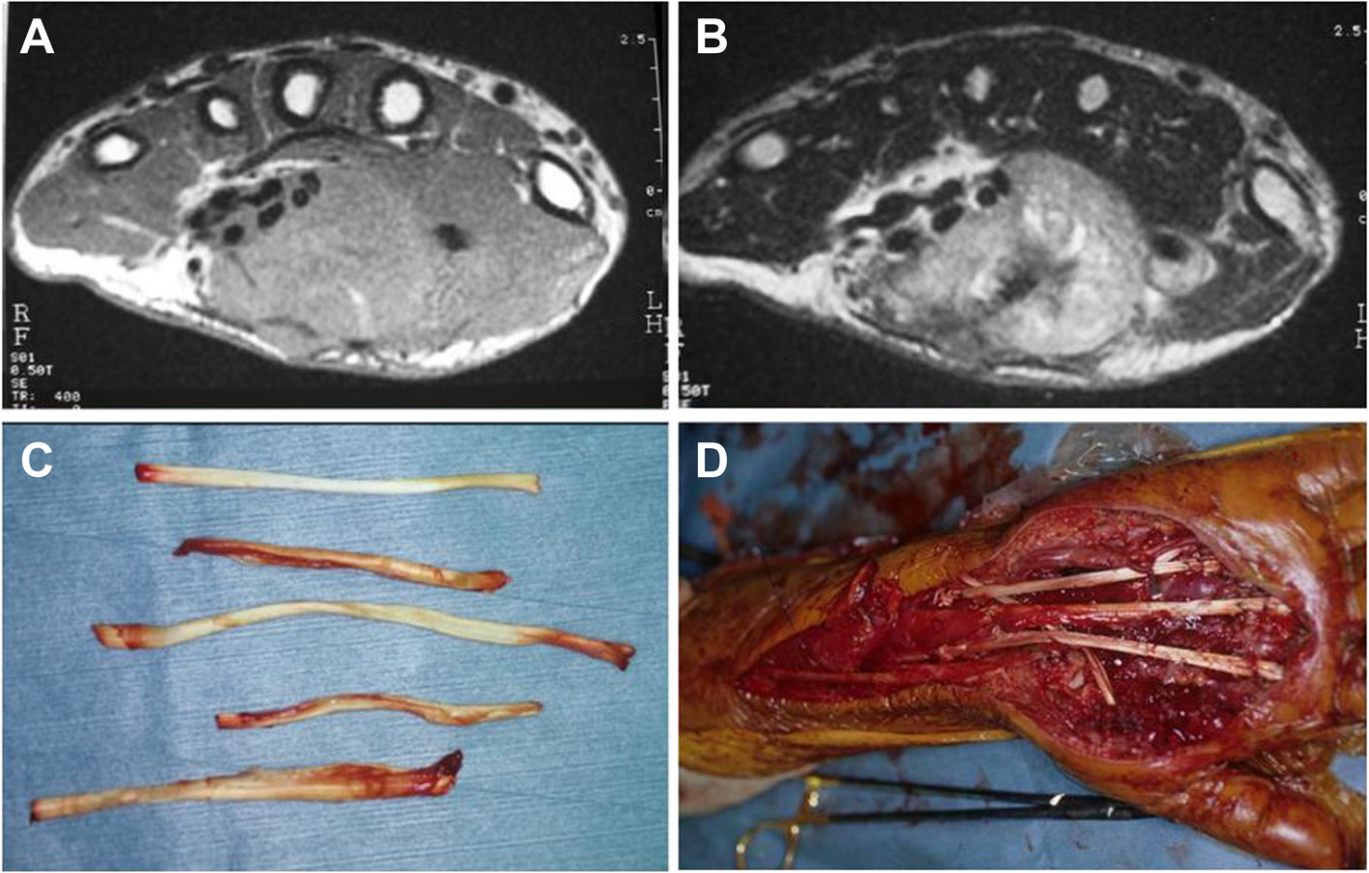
A 36-year-old male patient with clear cell sarcoma of the palm. MRI showed a mass that spanned the palmar aspect of the hand with iso-intensity on T1- (A) and was highly inhomogeneous on T2-weighted images (B) and involved the volar compartment (deep, superficial digital flexor tendon, and median nerve). The flexor tendons were stripped from the resected tumor tissues (C). The irradiated flexor digitorum profundus muscle was sutured to the opponens pollicis muscle, and skin flap was used for wound closure (D).
